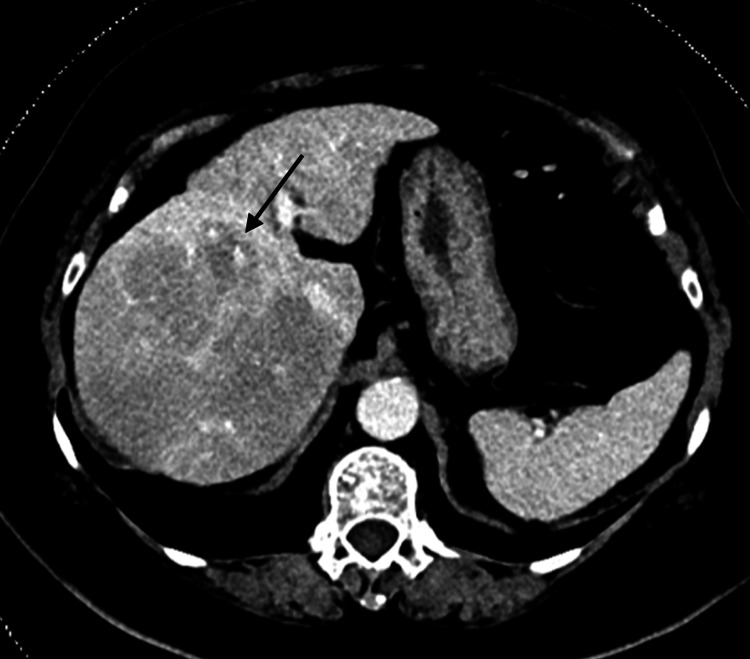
Contrast-enhanced CT abdomen and pelvis (June 2022): PV phase image The PV image shows a heterogeneous mass with margins centered in the right hepatic lobe (black arrow). It demonstrates relatively patchy hypoattenuation when compared with the left lobe of the liver and appears to have some degree of mass-effect CT: computed tomography; PV: portal venous
